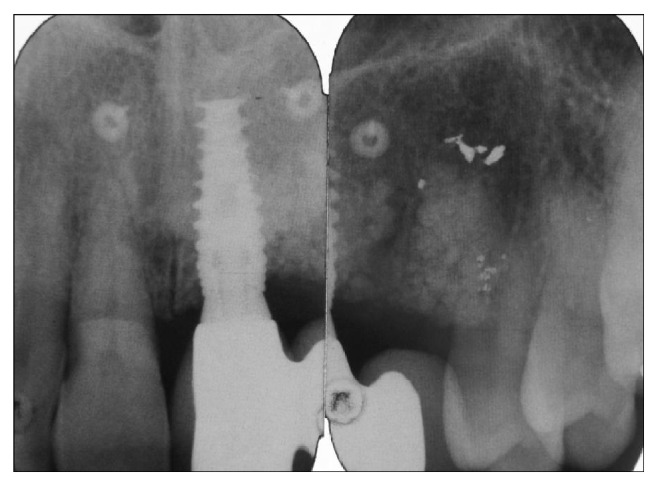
Periapical radiographs taken at initial consultation, showing a restored dental implant in position 21 (FDI-Notation) with no obvious signs of peri-implantitis. That restored implant carried a two-crown distal cantilever bridge.
